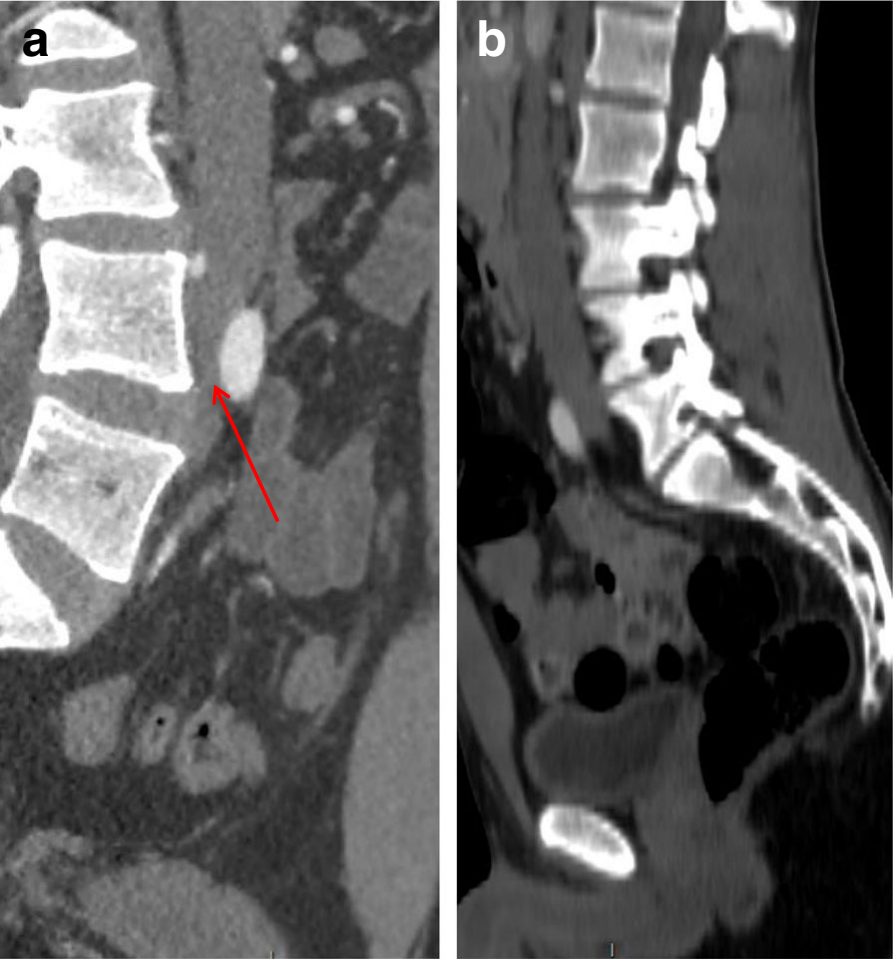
computed tomography angiography. a. Compression of the left common iliac vein between the right common iliac artery and lumbar spine. b. Normal left common iliac vein
